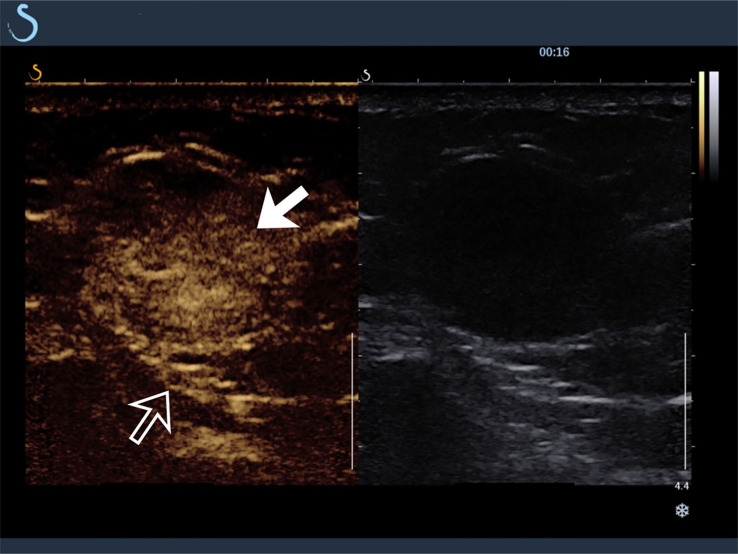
Contrast-enhanced ultrasound findings of mass in abdominal wall near the left waist (hollow arrow). The hypoechoic mass showed rapid hyperenhancement in arterial phase (solid arrow).
